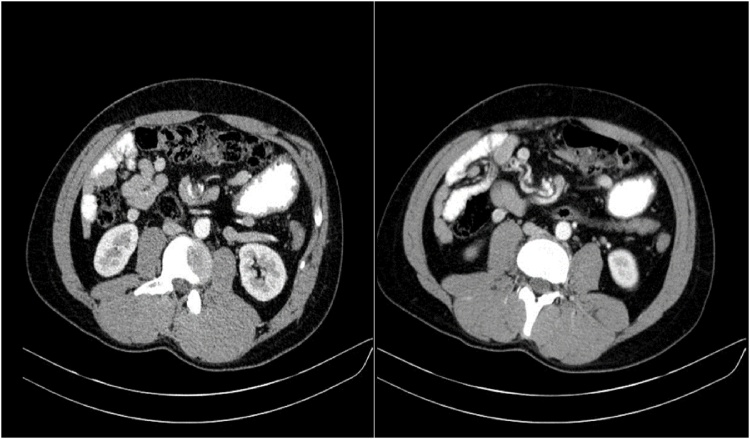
CT showing the characteristic whirpool’s sign.
